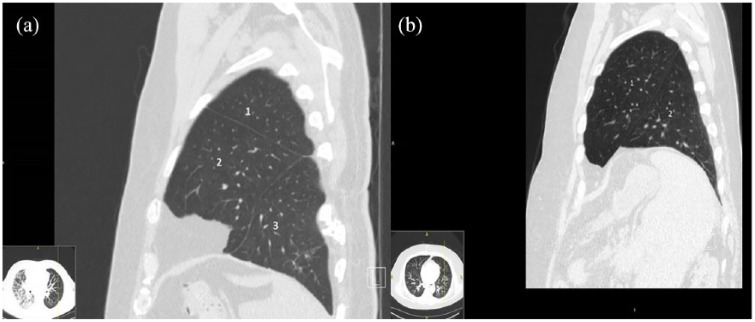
(a) Post-transplant computed tomographic image showing the three lobes of the left lung: 1, upper lob; 2, middle accessory lob bronchus; and 3, lower lob. (b) Normal variant of left lung: 1, upper lob and 2, lower lob.
